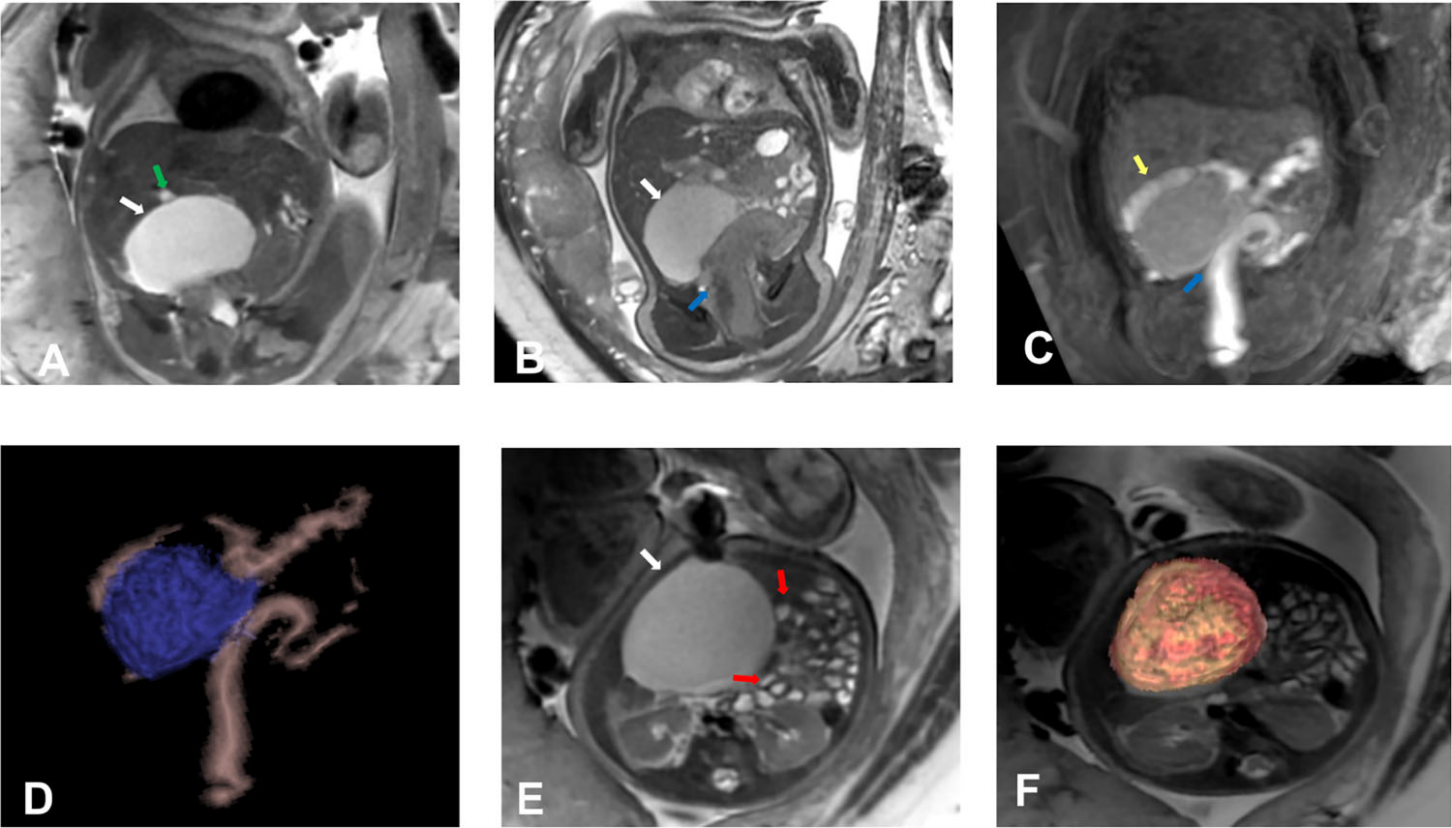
Right ovarian cyst. Fetal ultrasound: right hemorrhagic ovarian cyst. Gestational age 33 weeks and 3 days. A Coronal SS FSE T2. B Coronal FIESTA. C Coronal 3D LAVA. D 3D VR Coronal 3D LAVA. E Axial SS FSE T2. F 3D VR SSFSE. Intraperitoneal cystic lesion located in the right flank and right upper quadrant (white arrows) that slightly crosses the midline, indenting the hepatic flexure of the colon (yellow arrow), the inferior margin of the right hepatic lobe, and the gallbladder (green arrow). It displaces the loops of the small intestine to the left (red arrows) and contacts the distal sigmoid colon (blue arrows). It measures 53 mm in the transverse axis, 36 mm in the craniocaudal axis, and 48.5 mm in the anteroposterior axis
